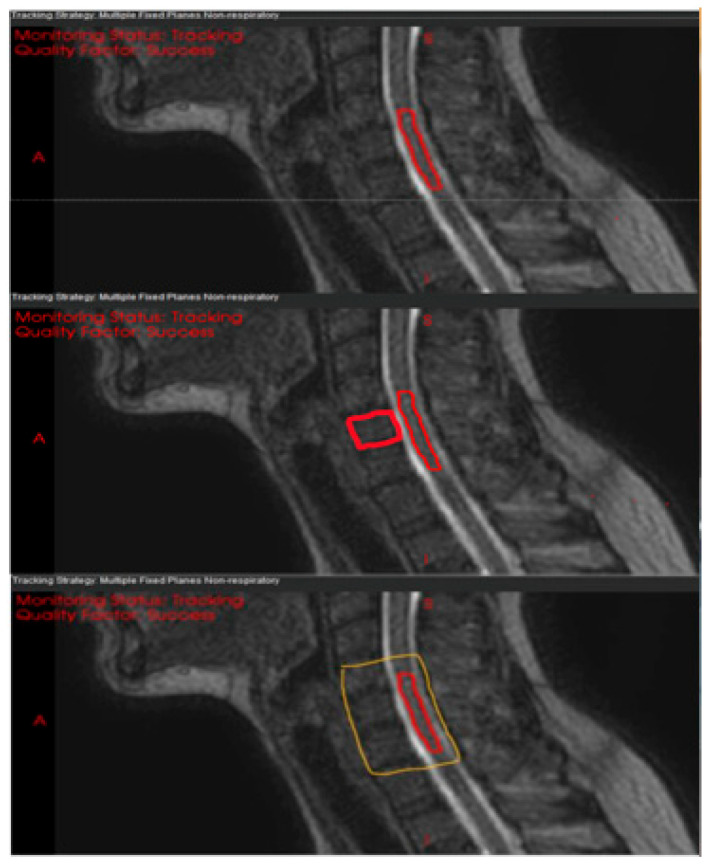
Three candidate registration structures for motion tracking on sagittal MRI scans: (top), spinal cord only; (middle), clinical target volume (CTV) plus cord; (bottom), spinal canal plus three adjacent vertebrae.
